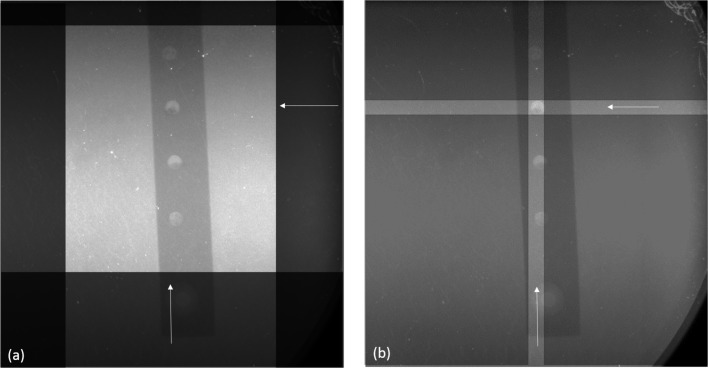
X-ray radiographs of the MCE in the well of the electrochemical cell showing *X–Y* alignment. (*a*) The MCE with the slits open and (*b*) complete alignment of the UO_2_-filled hole.
